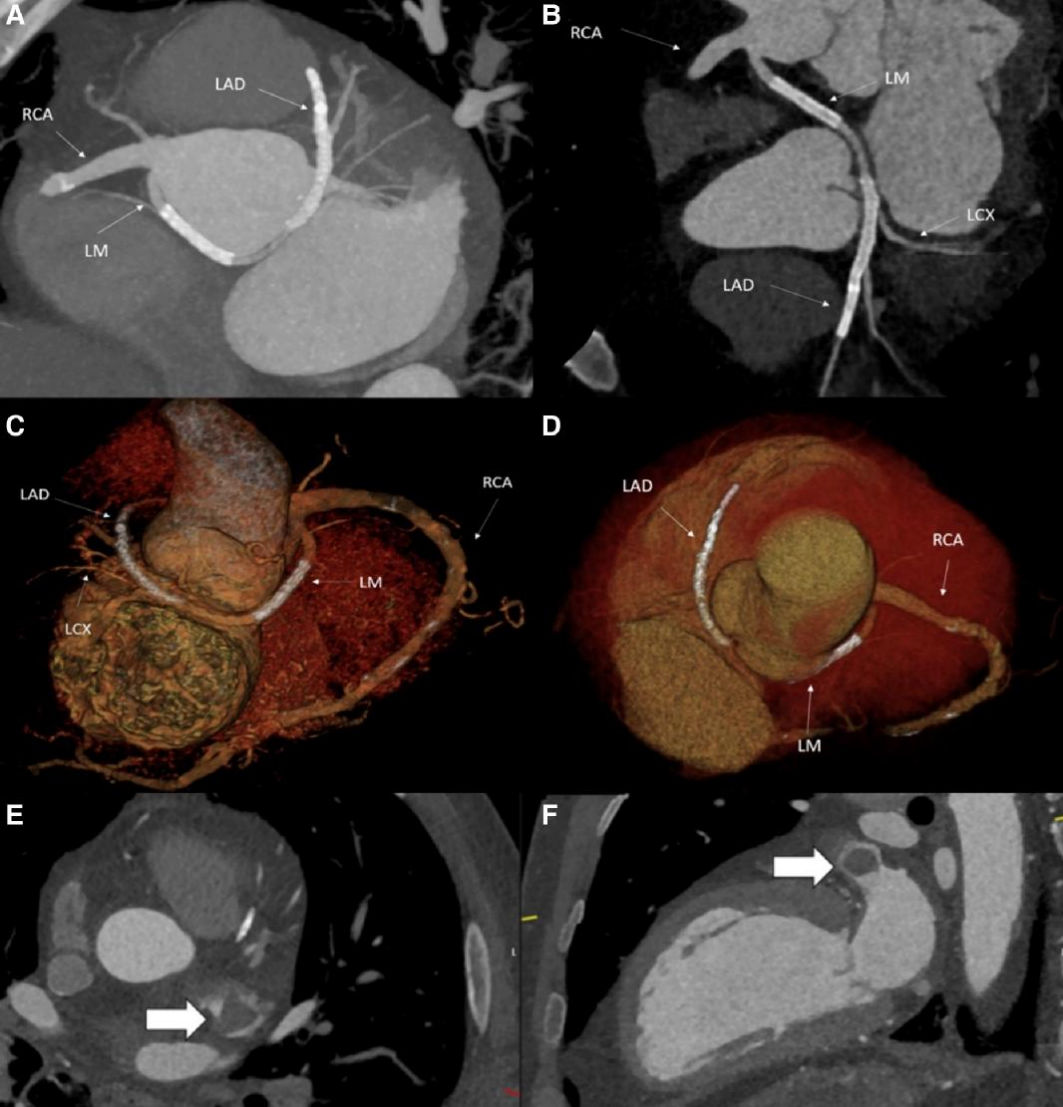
Computed tomography angiography. (*A* and *B*) Multi-planar reconstruction images showing origin of the anomalous left main coronary artery from the right coronary sinus, with stents in the left main coronary artery and left anterior descending artery, and the absence of intramural course, acute angulated take-off, or slit-like narrowing. (*C* and *D*) 3D volume rendering images showing retroaortic course of the left main coronary artery. (*E* and *F*) Large filling defect (indicated by block arrows) in the left atrial appendage indicative of a thrombus.
